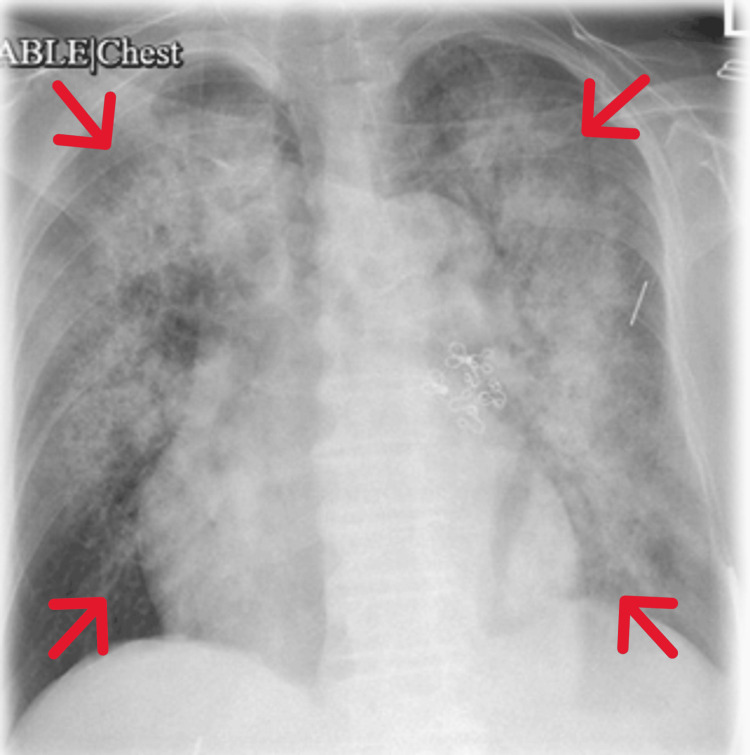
Portable AP chest radiograph Demonstrating bilateral pulmonary infiltrates (red arrows) consistent with diffuse alveolar hemorrhage.
